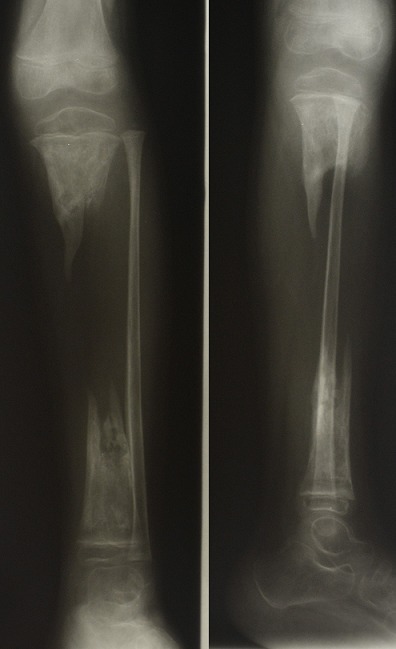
Lateral and PA radiograph of the right tibia and fibula post sequestectomy showing a large segmental bone defect
